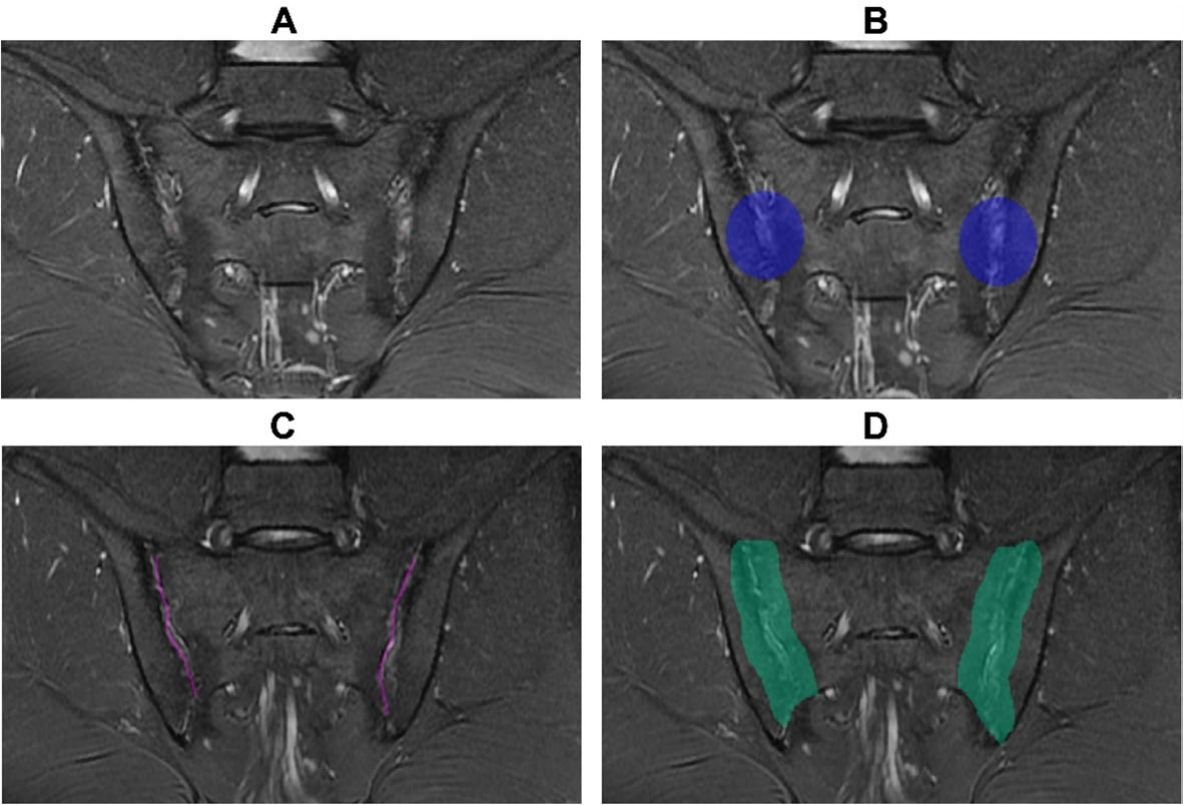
SIJ-MRI segmentation and expansion for four ROIs: CircleOriginal (A), Circle (B), Facet (C), and FacetOriginal (D). MR, magnetic resonance; SIJ, sacroiliac joint
